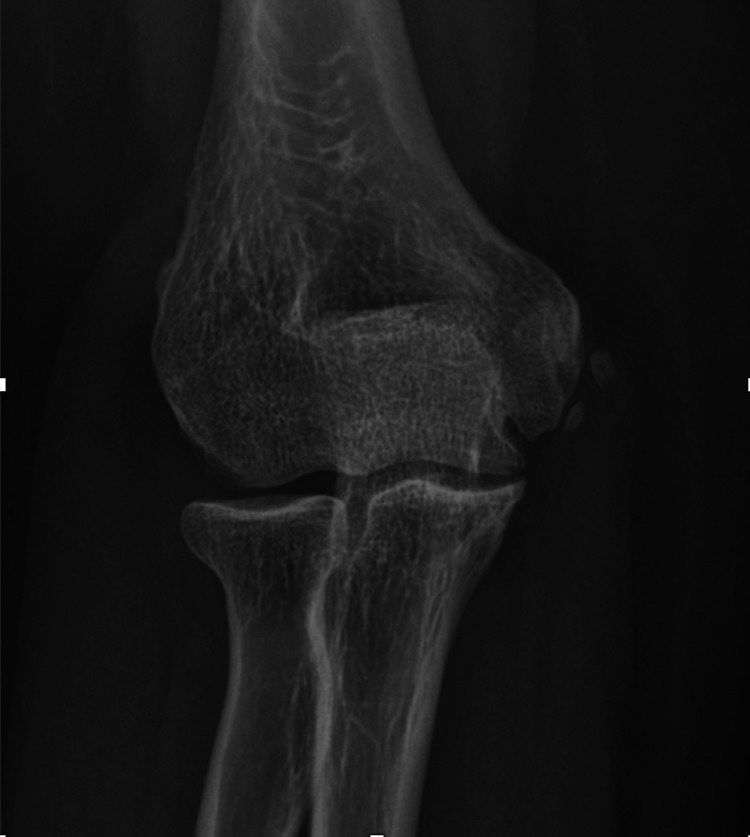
Elbow X-ray showing signs of degenerative disease with osteophyte formation, joint space reduction, and medial periarticular ossifications that compress the UN
